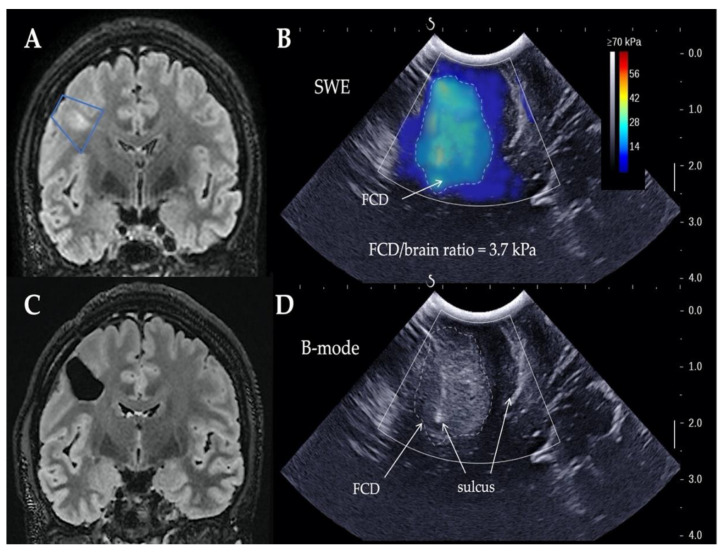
(A) Preoperative and (C) postoperative magnetic resonance imaging (MRI) images (T2-weighted FLAIR, coronal views) in patient with MRI-positive right frontal focal cortical dysplasia (FCD). Blue trapezium: ultrasound imaging plan. (B) Intraoperative elastography and (D) ultrasound B-mode images. Dimensions of ultrasound images: 38.4 mm wide and 40.0 mm deep. (B) Zone of increased stiffness (dotted line) highlighted FCD, and (D) B-mode image showed abnormal hyperechogenicity. SWE, shear wave elastography.
